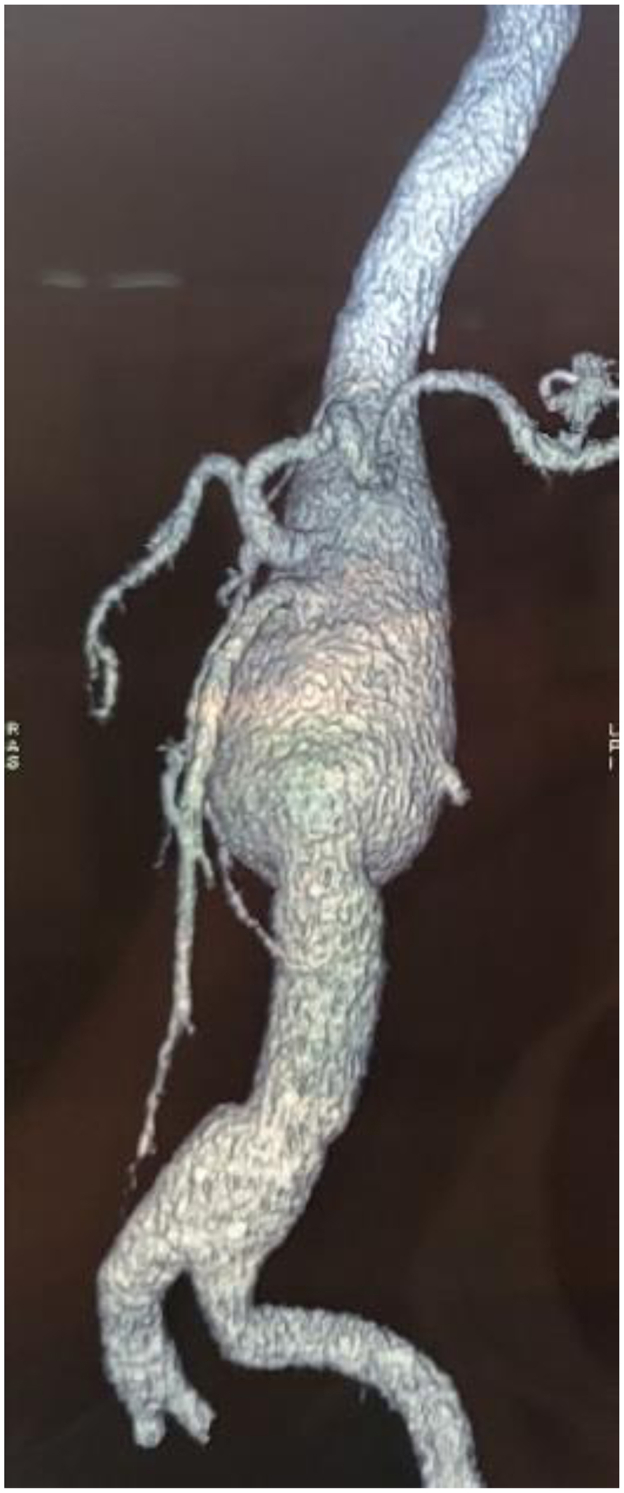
Six-month postoperative angio-computed tomography (CT) scan showing a patent graft and significant enlargement of the visceral aorta above the reconstruction.
